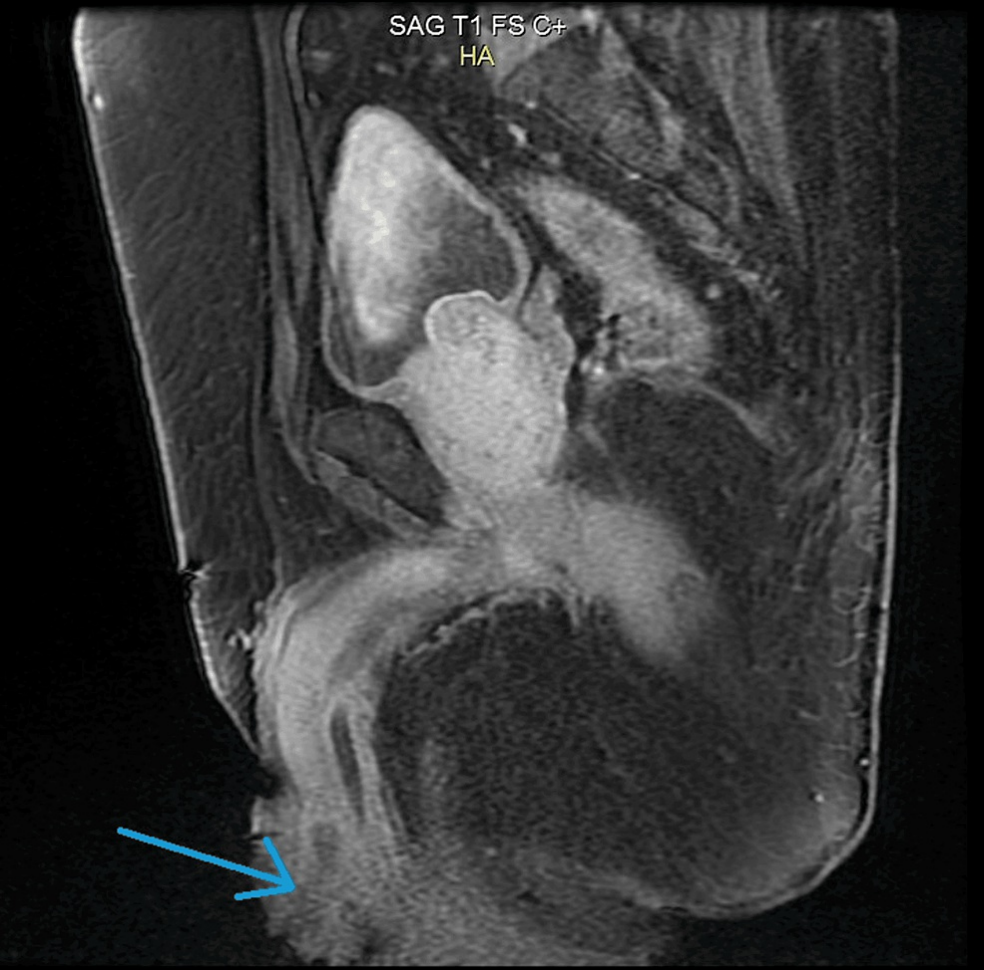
Sagital pelvic MRI T1 showing tumor thickening centered on the glans of the penis (blue arrow), infiltrating the fascia and the spongy urethra
